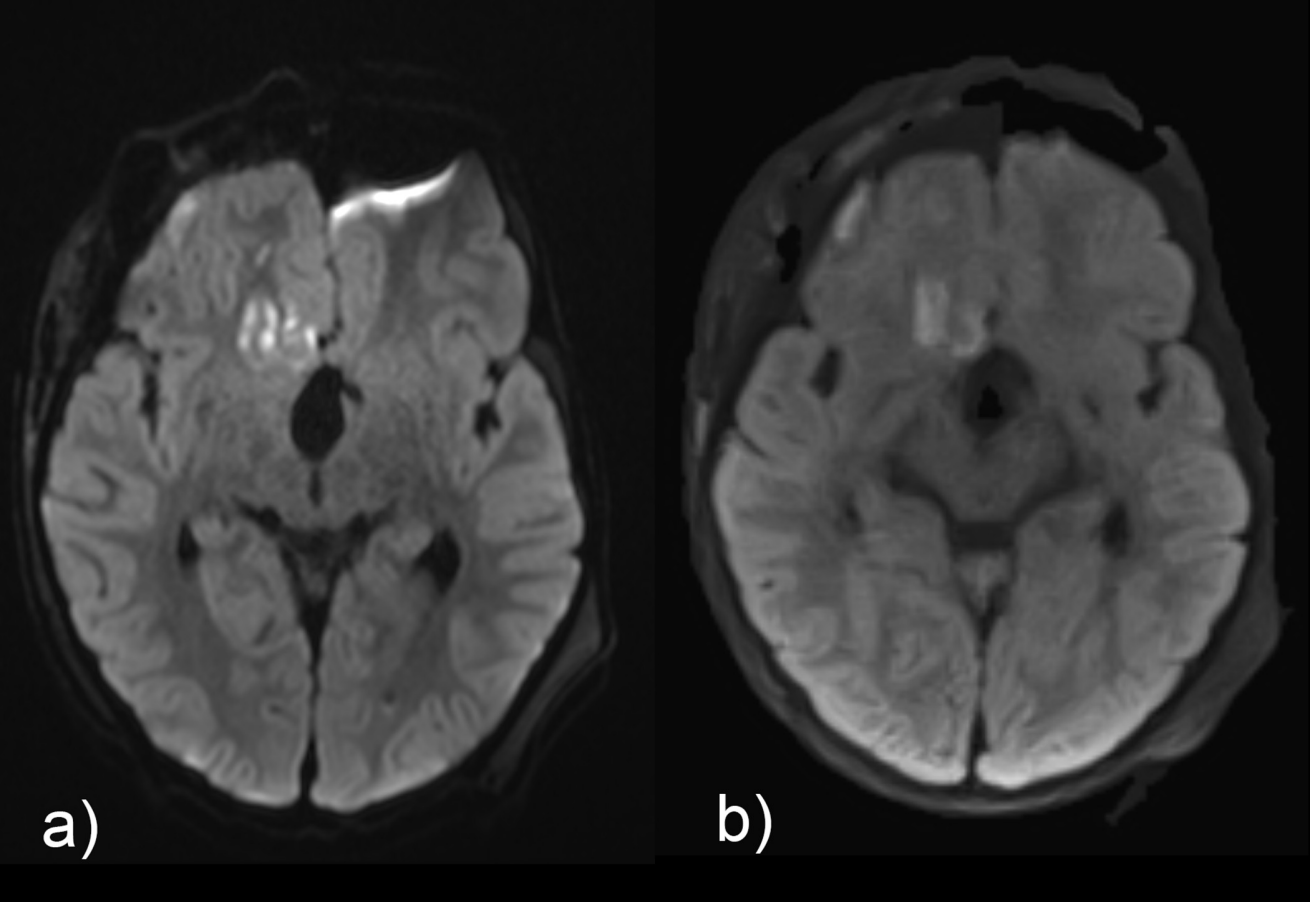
Comparison of isoDW images obtained by (a) EPI-DWI and (b) STEAM-DWI in a 4-year-old boy with status post resection of craniopharyngeoma. In contrast to EPI-DWI, the left-frontal pneumencephalus does not cause artifacts with STEAM-DWI. Please note the hyperintense changes in isoDWI which showed restricted diffusion in ADC, in the right hemisphere in both the fontal cortex and frontal white matter.
